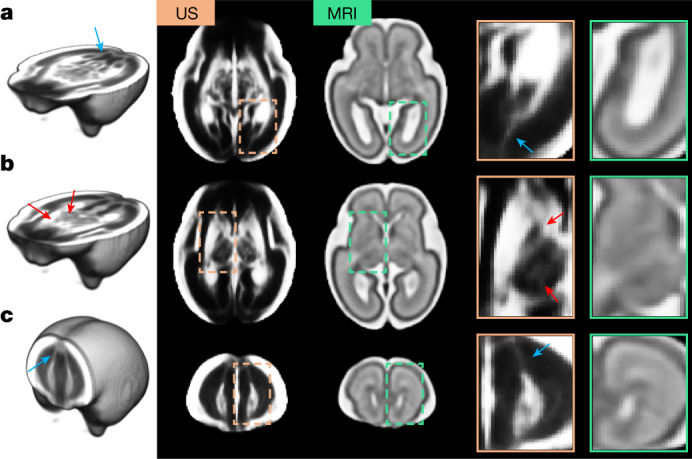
Comparison between US and MRI atlases of the fetal brain. a–c, Age-matched templates shown at two axial views: level of the ventricles (a) and level of the thalami (b), and one coronal view (c). Structures colocalize in both modalities, but US templates showed sharper tissue boundaries in the subcortical grey matter areas (red arrows). Blue arrows indicate possible white matter fibre bundles in the US atlas, which are not visible in the MRI atlas. Note that the contrast of the US atlas has been edited to highlight the key structures.
